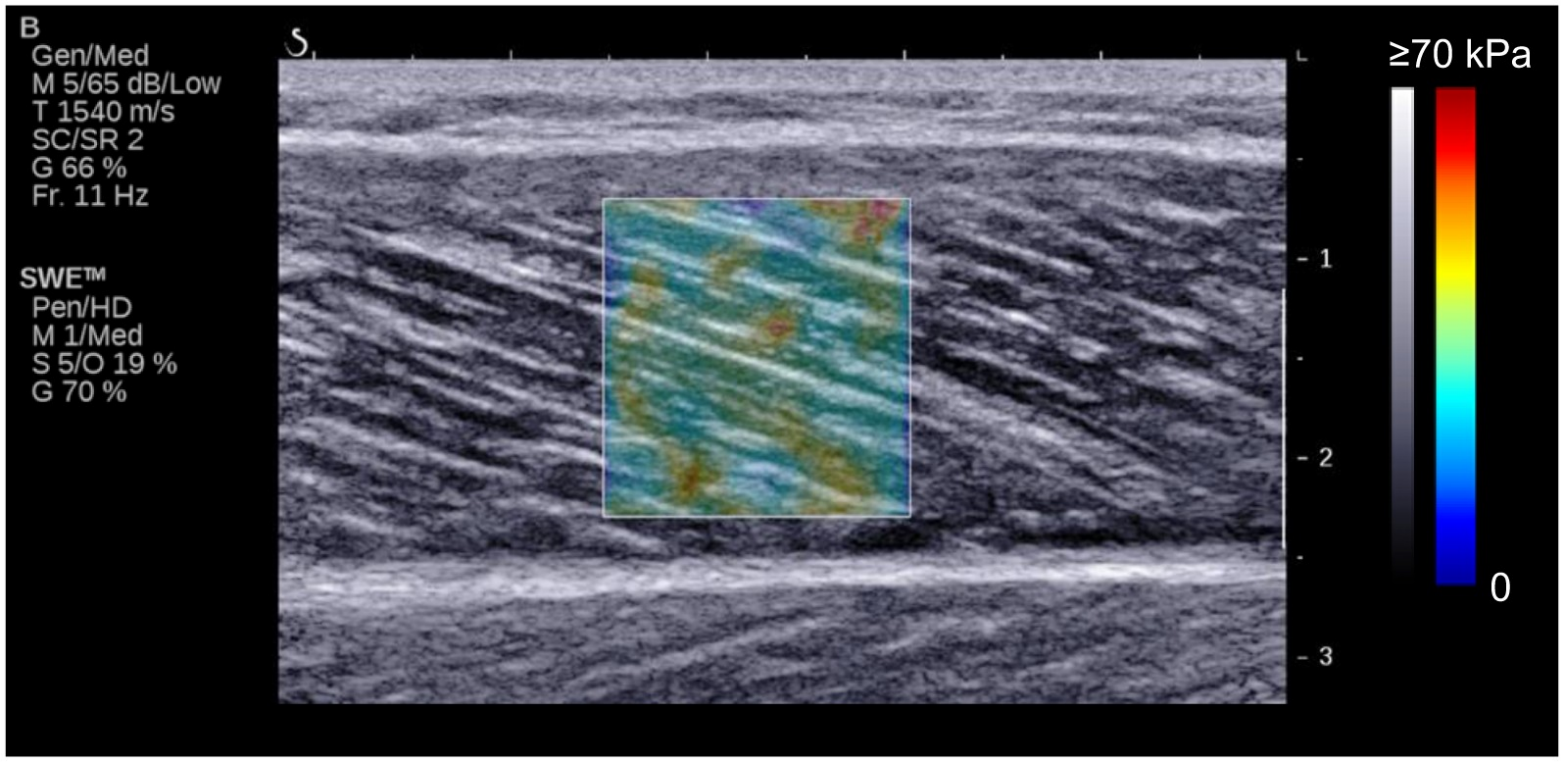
Typical example of ultrasonic image. Colored square region represents shear modulus map with the scale to the right of the figure.
